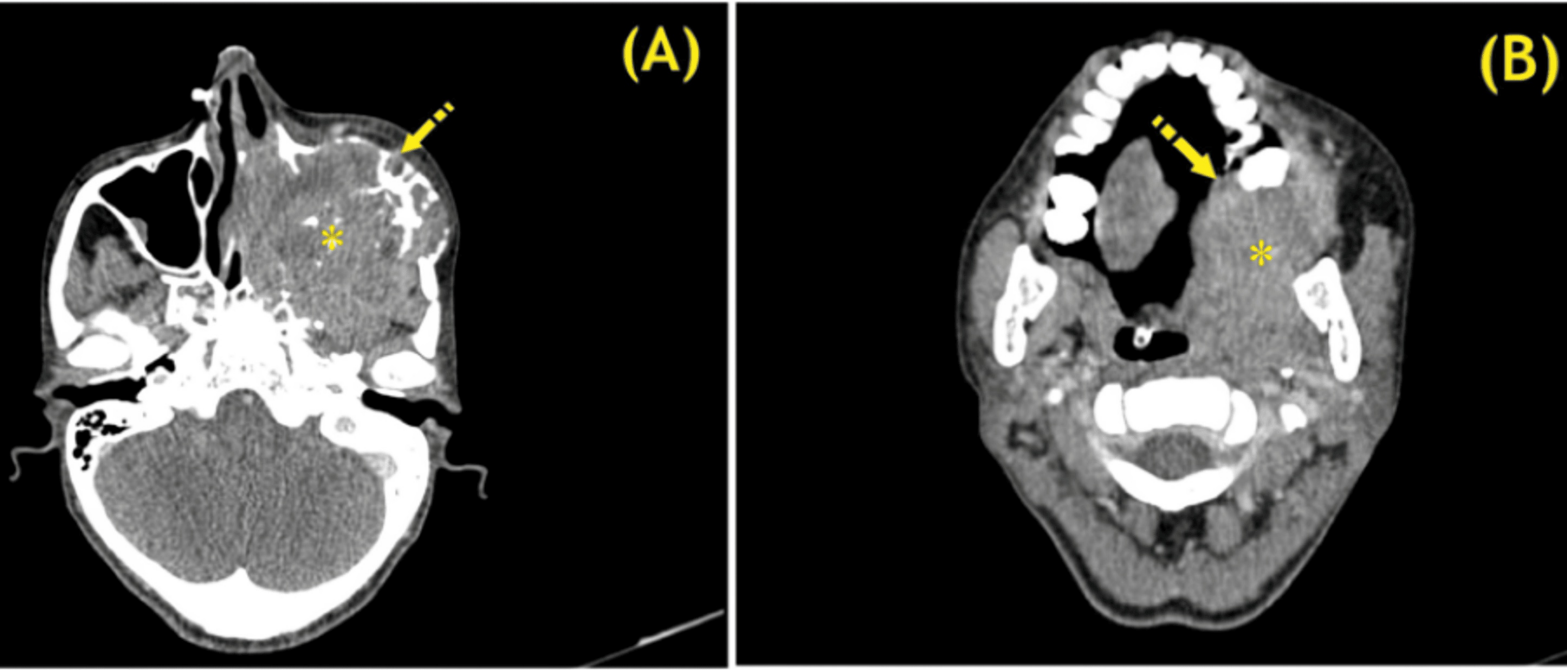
(A) Axial CECT scan of the head and neck showing a large, heterogeneously enhancing mass (asterisk) occupying the nasal cavity and left maxillary sinus, with destruction of the medial wall of the left maxillary sinus, and partial destruction and bowing of the anterior and lateral maxillary sinus walls (arrow); (B) Axial CECT scan showing inferior tumor extension (asterisk), causing dehiscence of the hard palate and intraoral protrusion on the left side (arrow). CECT, contrast-enhanced computed tomography
